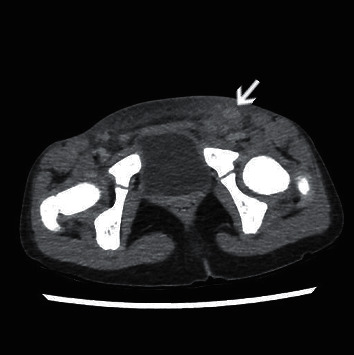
Enhanced computed tomography findings. A pelvic enhanced computed tomography scan showing an enlarged left inguinal lymph node (white arrow, approximately 1.5 × 1.0 cm), densification, and thickening of the regional soft tissue without abscess.
